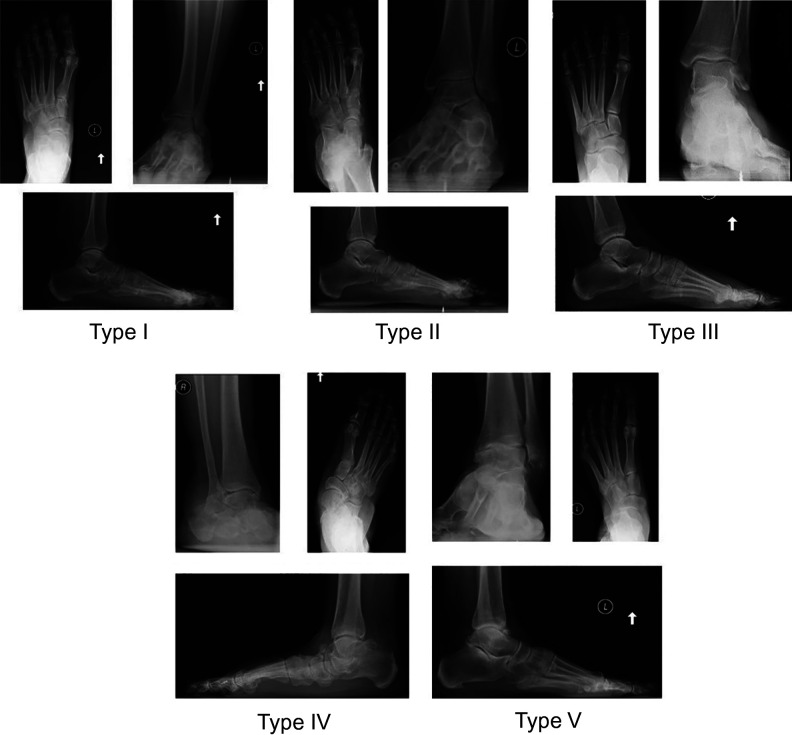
Radiographic examples of each of the five types of the Grand Rapids Arch Collapse Classification.
